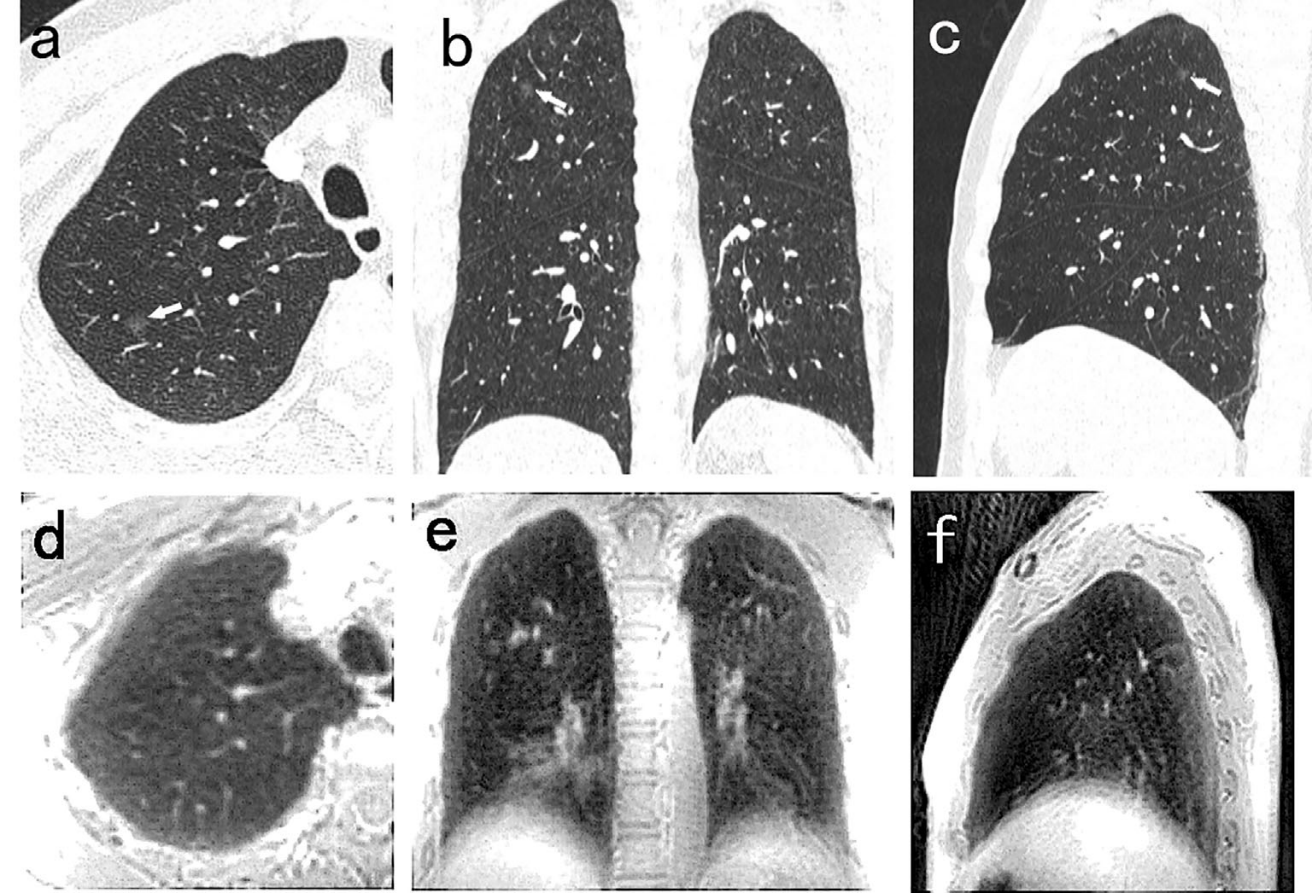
A 54-year-old man with 5.5mm GGN (arrow) in the right upper lobe. The nodule is well visualized on the CT axial (A), coronal (B), and sagittal (C) image, and is not detected in the PETRA sequence (D–F). The arrows represent the locations of nodules.
